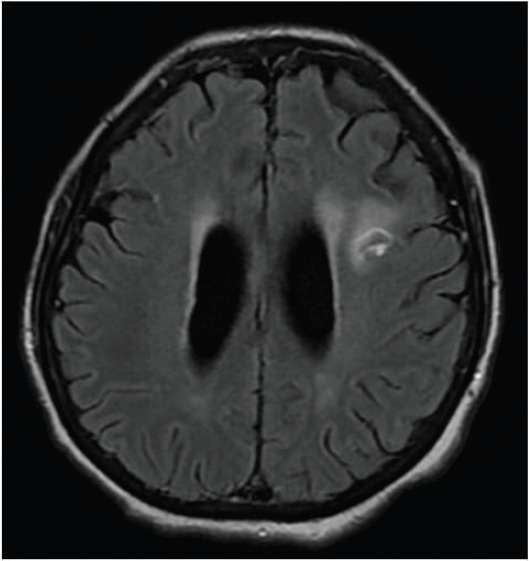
Reduction in size of the ring-enhancing lesion and resolution of oedema (compared to Figure 2). A follow-up MRI performed after 3 weeks of treatment showed interval reduction in size of the ring-enhancing lesion and resolution of the oedema (as compared to Figure 2). The other smaller lesions were also less well appreciated on this study.
